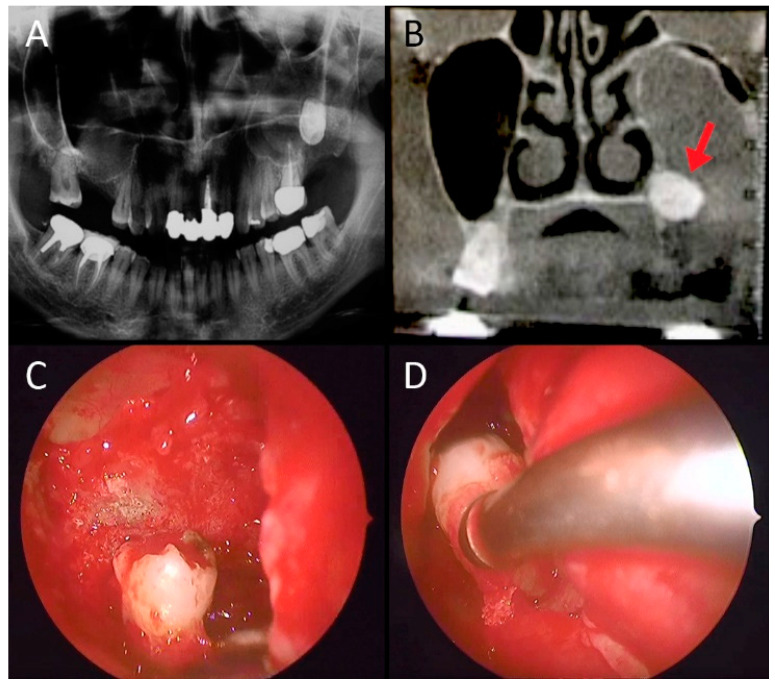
Male, 46 years old, complaining of nasal obstruction, rhinorrhea and foul odor and taste, without dental symptoms. The panoramic X-ray (A) and the CT scan (B) revealed a large odontogenic cyst of the left maxillary sinus, with an impacted tooth inside (red arrow in (B)). An exclusive endoscopic endonasal surgical approach was chosen; the tooth (C) was therefore removed (D) together with the cyst endoscopically.
